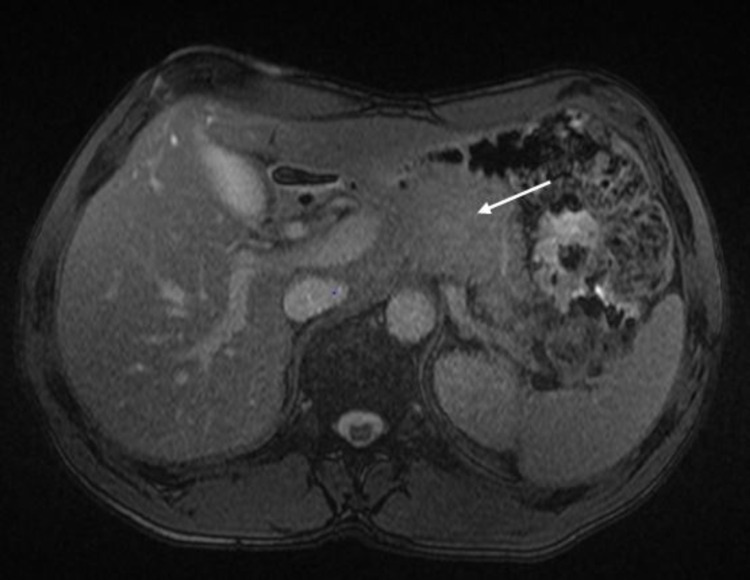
MRI abdomen showing a 44 x 31 mm, hypo-enhancing mass (arrow) in the pancreatic tail.
